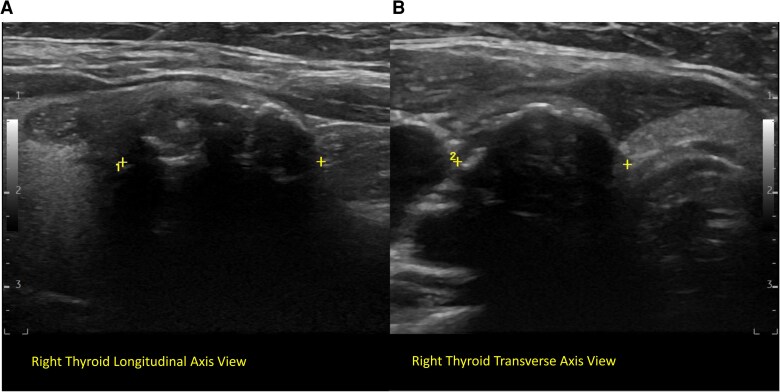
Thyroid and neck ultrasound: longitudinal axis view (A) and transverse axis view (B) images demonstrating a densely calcified right thyroid nodule with marked shadowing and measuring 2.1 × 1.8 cm. This nodule was biopsy-proven papillary thyroid carcinoma.
